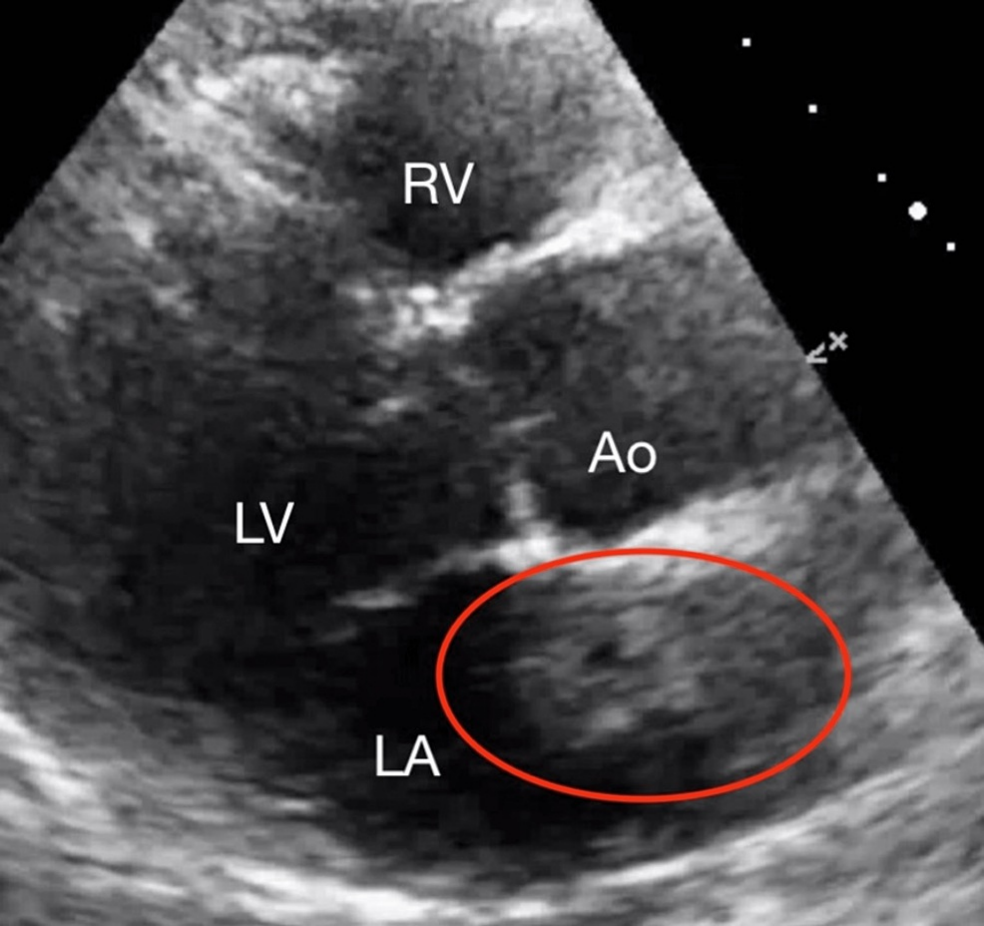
Parasternal Long-Axis View of Atrial Myxoma Transthoracic echocardiogram demonstrating a partially obstructive mass (circled) tethered to the interatrial septum LA = left atrium; LV = left ventricle; RV = right ventricle; Ao = aorta
